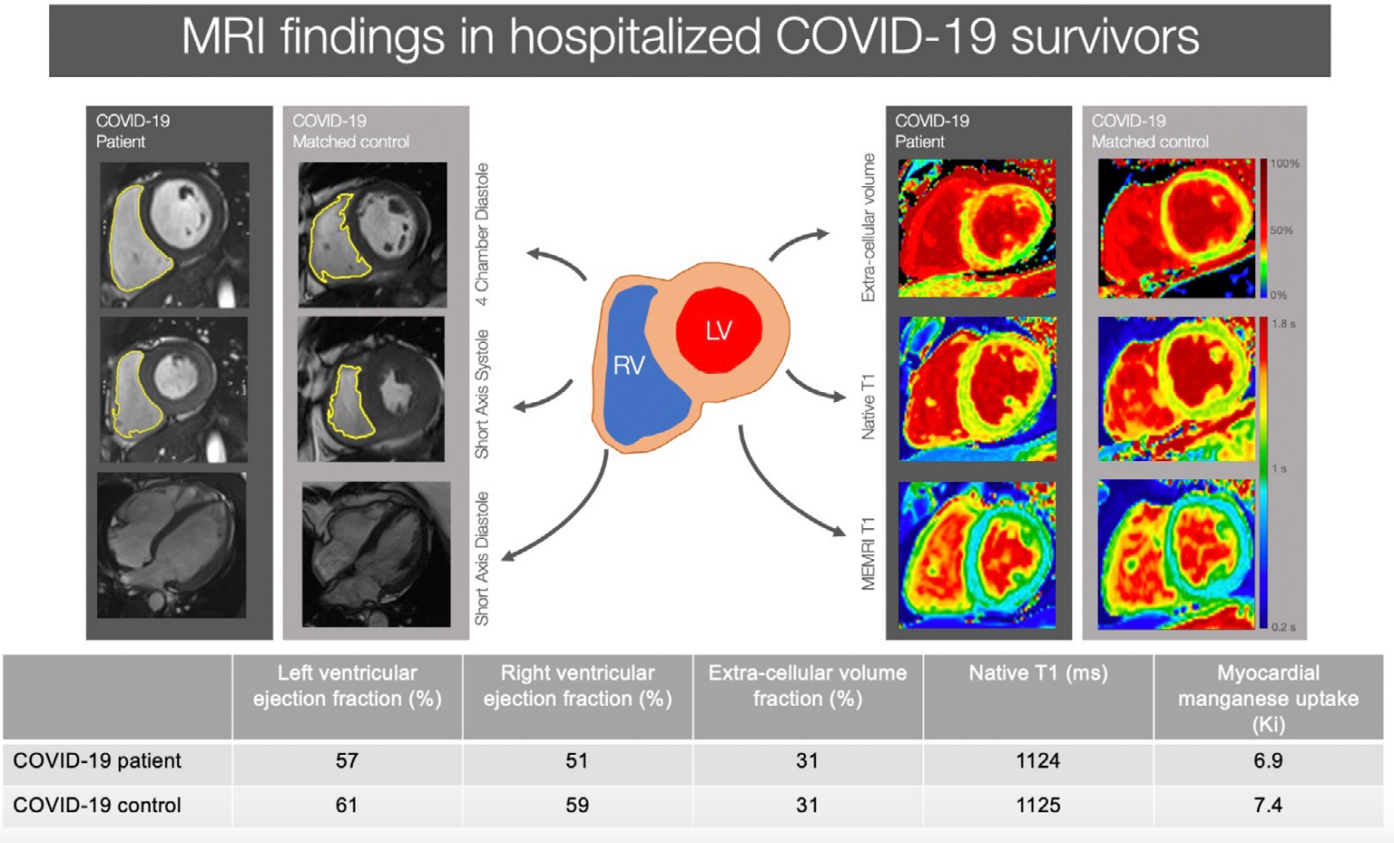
Cardiac magnetic resonance features of patients hospitalized with coronavirus disease‐19. Magnetic resonance imaging findings in patients recovering from COVID‐19 infection compared to age‐, sex‐, and co‐morbidity‐matched volunteers. MEMRI = manganese‐enhanced magnetic resonance imaging; LV = left ventricle; RV = right ventricle.
